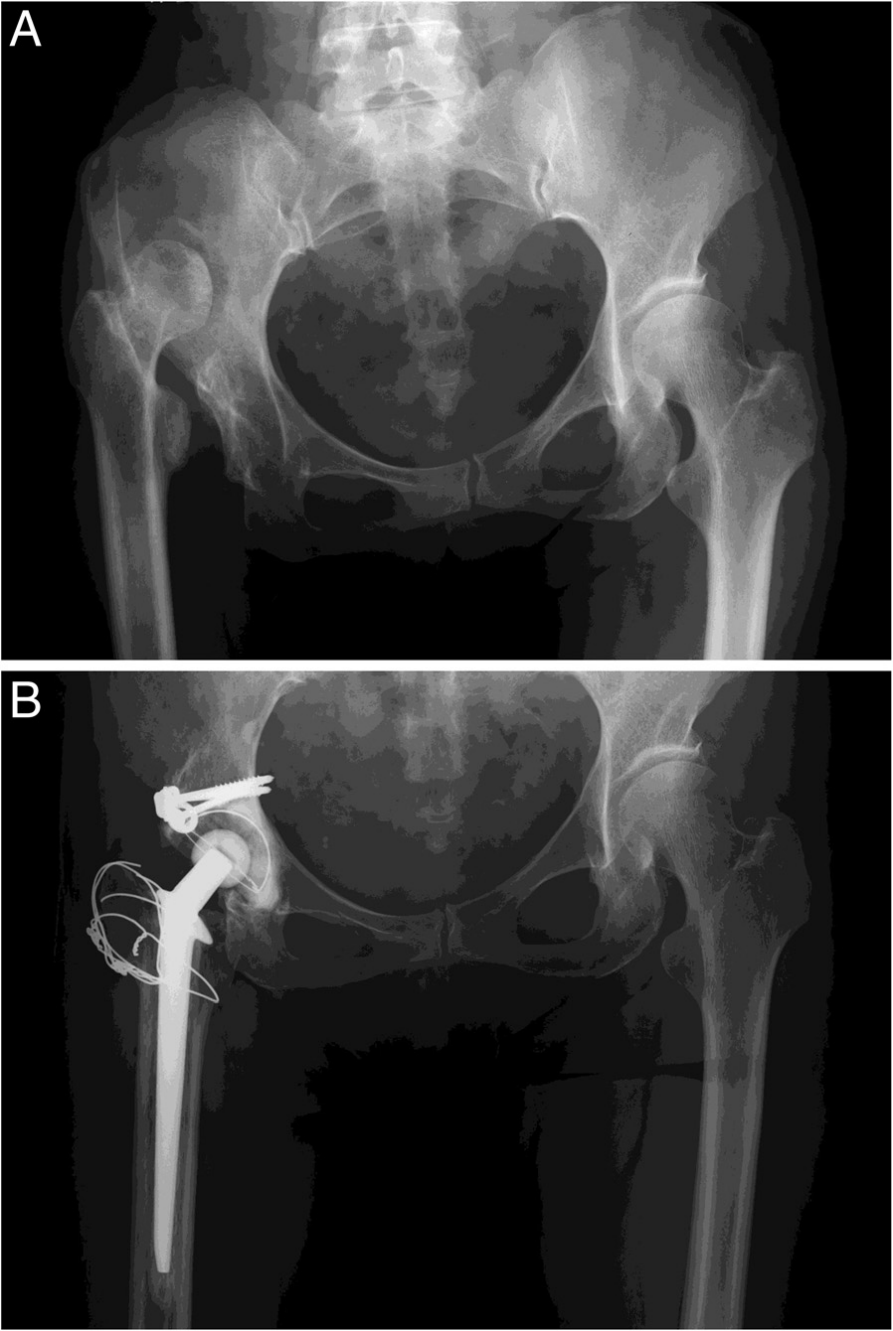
Radiographs of a representative case. A. An anteroposterior pelvic radiograph of a 57-year-old woman with Crowe IV complete dislocation of the right hip. B. A postoperative radiograph at 11 and a half years after a total hip arthroplasty with limb lengthening by 4.5 cm, showing no loosening or radiolucent line.
